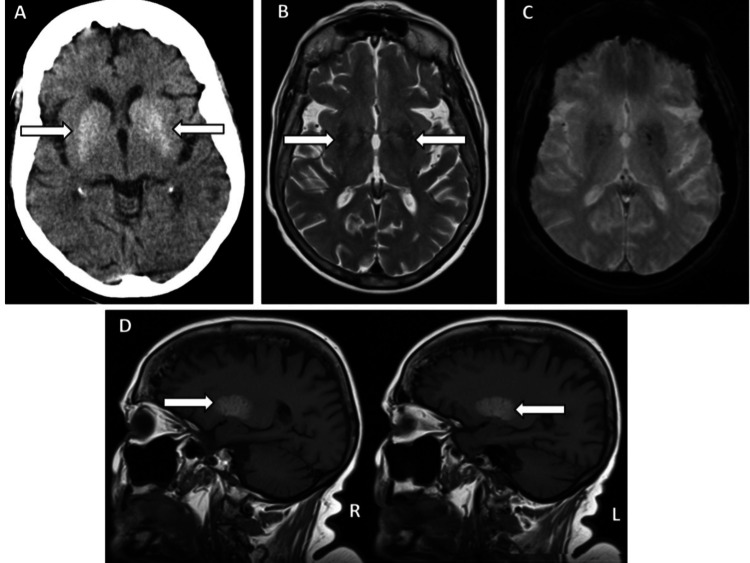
Brain images of case 2. A. Axial noncontrasted brain CT scan on admission demonstrates bilateral, symmetric, homogeneous hyperattenuation of lentiform and caudate nuclei. This density alteration respects the expected anatomy being confined to the basal ganglia without marked distortion. No signs of mass effect or edema. In the remaining parenchyma, there were no signs of acute intracranial lesions.
